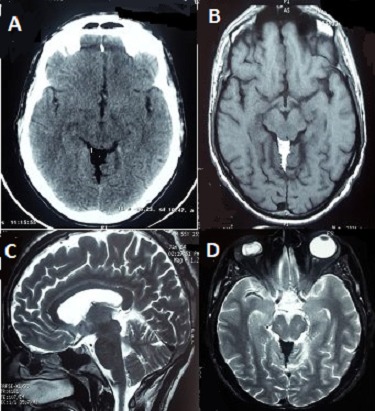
(A) CT scan showing a hypodense lesion in quadrigeminal plate cistern. (B,C,D) MRI scan, T1 imaging axial view: (B) T2 imaging sagittal view; (C) axial fat-suppression sequence; (D) quadrigeminal cistern lipoma
